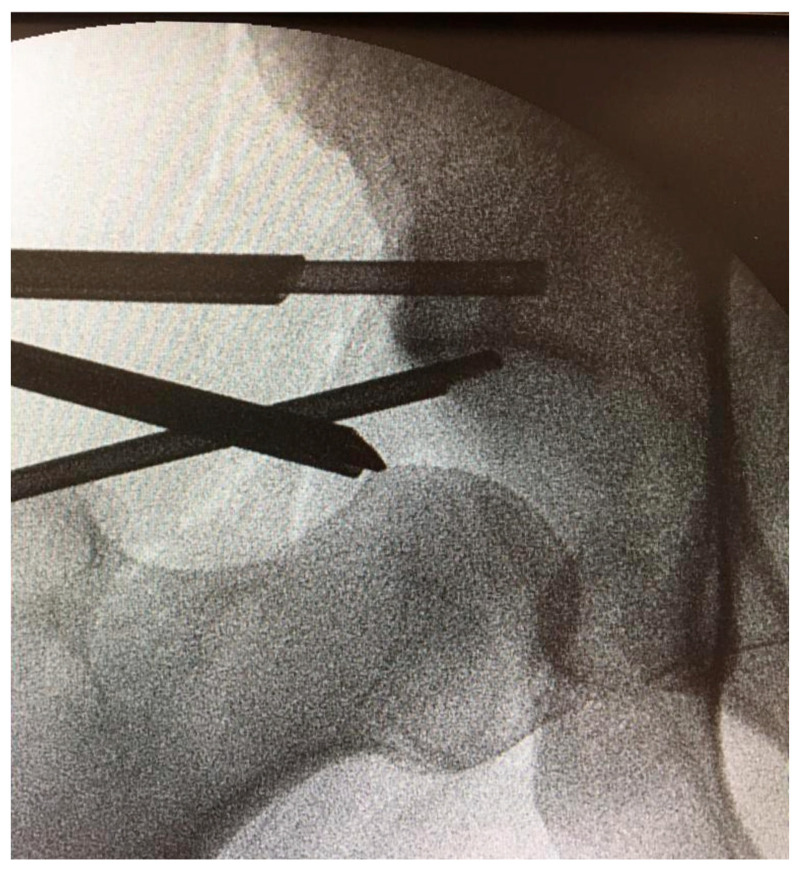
Bone marrow lesion targeting for correct AccuFill^®^ injection by using orthogonal fluoroscopic views that matched the associated MRI sliced.
